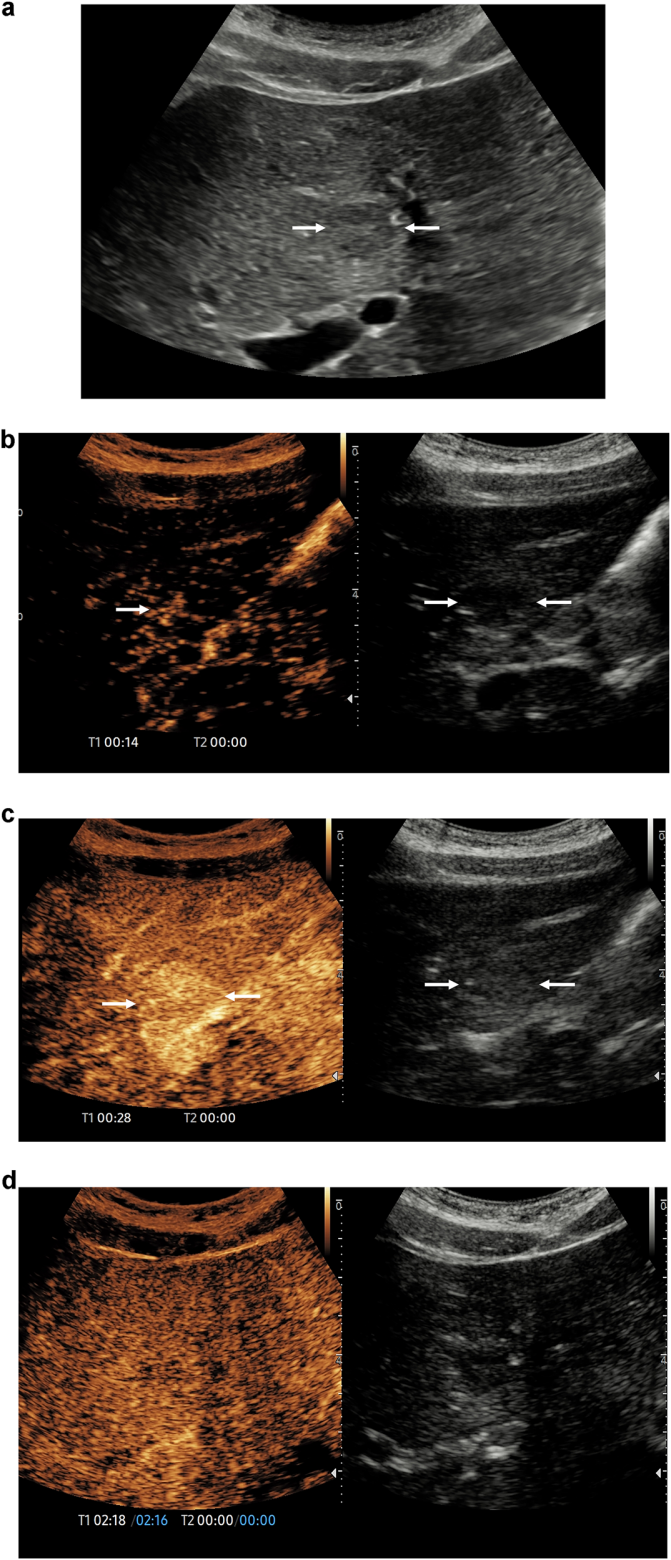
A 14-year-old female patient with chronic liver disease, a consequence of autoimmune hepatitis, found to have a new focal lesion on a liver ultrasound examination, and demonstrating indeterminate ultrasound features. a A focal 12 × 11 mm low reflective liver lesion(arrows) on a background of a heterogenous liver. b Following the administration of 2.4 mL of SonoVue™ (Bracco SpA, Milan), during the arterial phase at 14 s, there is a central spoke wheel arterial pattern (single arrow), in the focal liver lesion (arrows). This raises the possibility of the presence of an area of focal nodular hyperplasia. c At 28 s, during the early portal venous phase, the focal live lesion is hyper-enhancing (arrows) in comparison to the background liver parenchyma. d At 2 min 16 s, the late portal venous phase, there is similar washout to the background liver parenchyma, a feature of benign disease, and confirming the presence of an area of focal nodular hyperplasia
